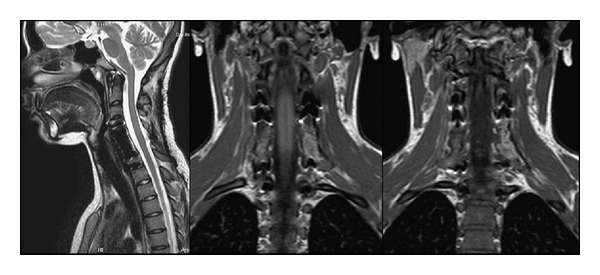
Five-year MRI scans, showing a complete regression of the extensive local recurrence.
